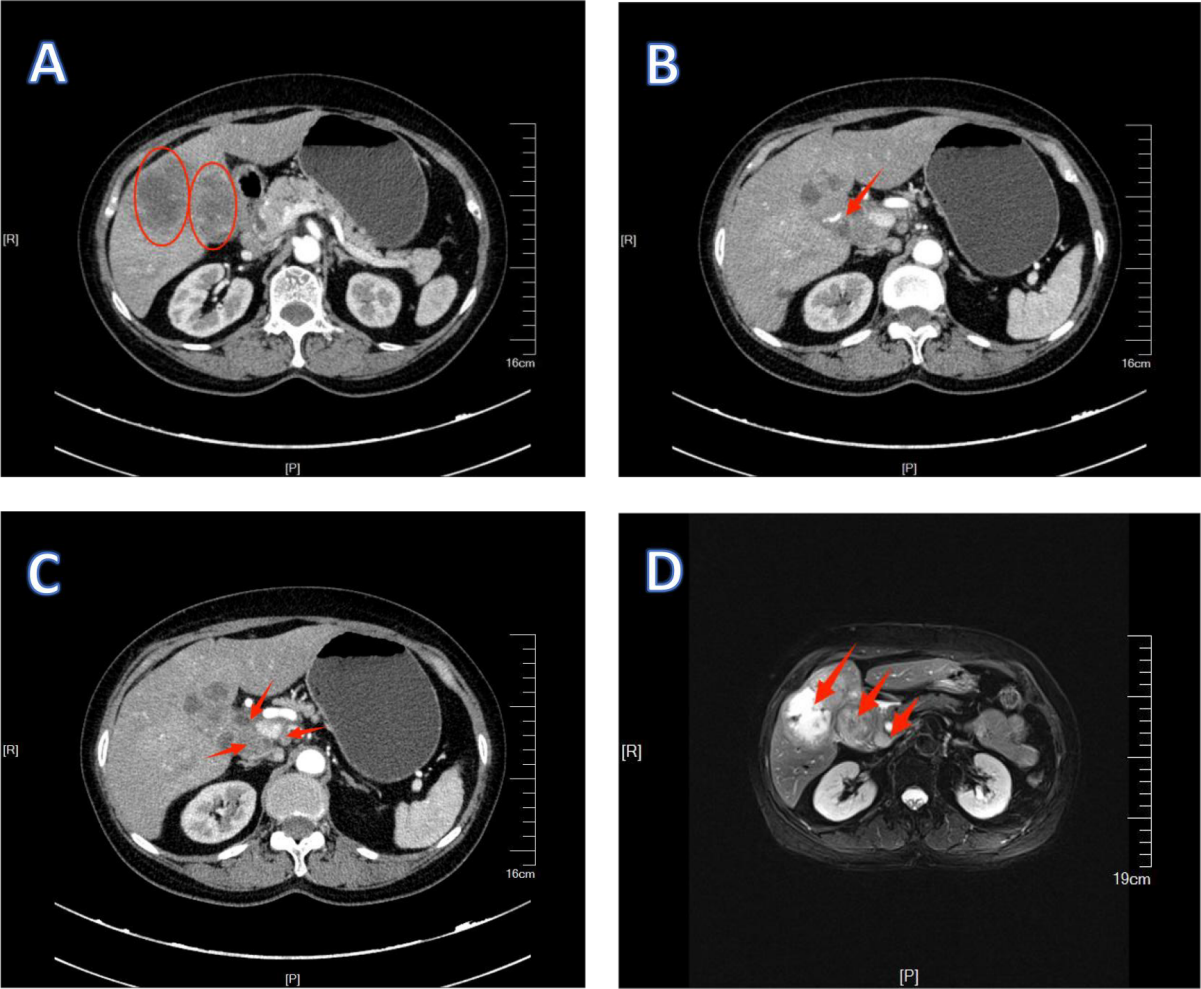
1Imaging findings of GBC in patient 2 without any treatment. (A–C) are CT images. (D) is an MRI image. (A) shows the primary lesion of gallbladder cancer and the infiltrating lesion in the right lobe of the liver. (B) shows that the lymph nodes surround the hepatic artery. (C) shows lymph node metastasis around the hepatic hilum.
